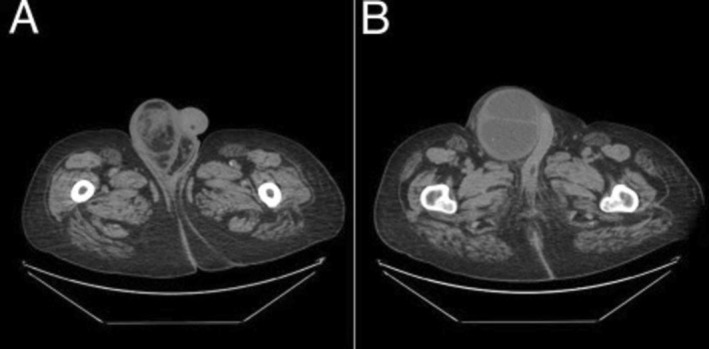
(A, B) Computed tomography of the abdomen and pelvis without contrast showing a large right inguinal hernia.
